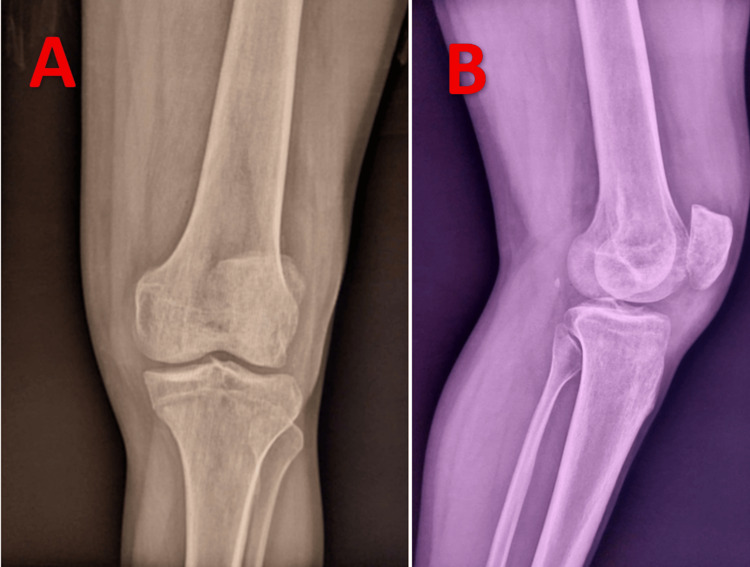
Standard anteroposterior (A) and lateral (B) radiographs showing no abnormalities.
